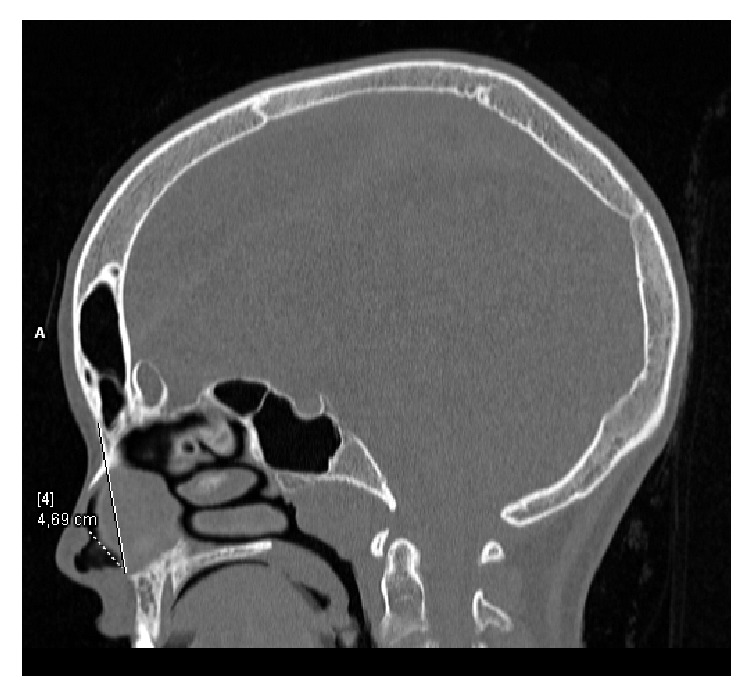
An example of n/ns distance marked on the CT image.
